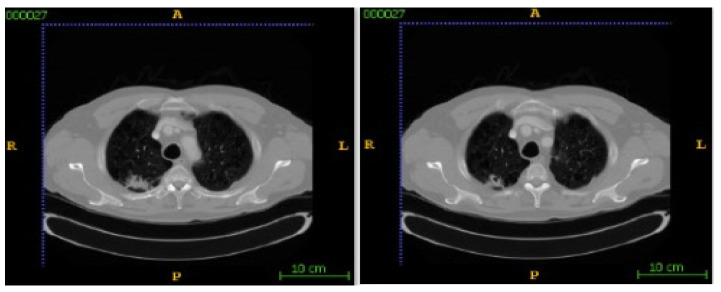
CT slices showing juxta-vascular nodules (Patient R_0052).
